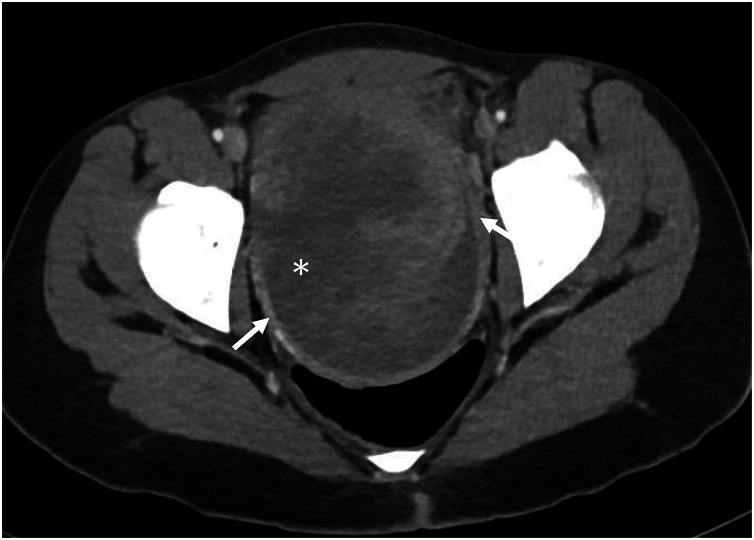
Contrast-enhanced axial computed tomography (CT): large peripherally enhancing (arrows) heterogeneous cervical mass with central fluid attenuation representing hemorrhagic component (asterisk).
